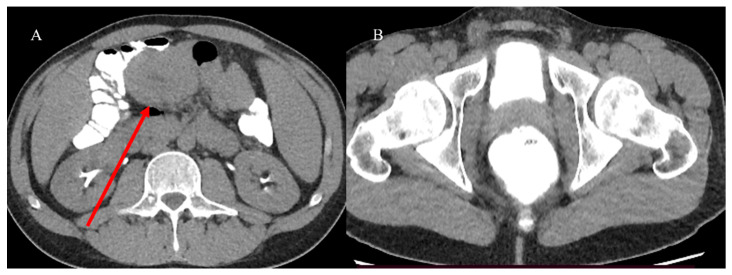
The CT scan shows integration with GGF in the previous case of a 28-year-old man with appendicitis and IS. The invagination was partially resolved (red arrow) in image (A), and the GGF progressed in the rectum (B).
